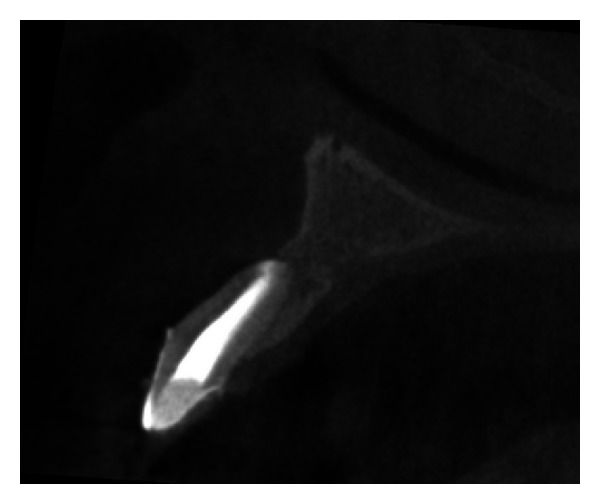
1-year followup CBCT image in sagittal plane.
